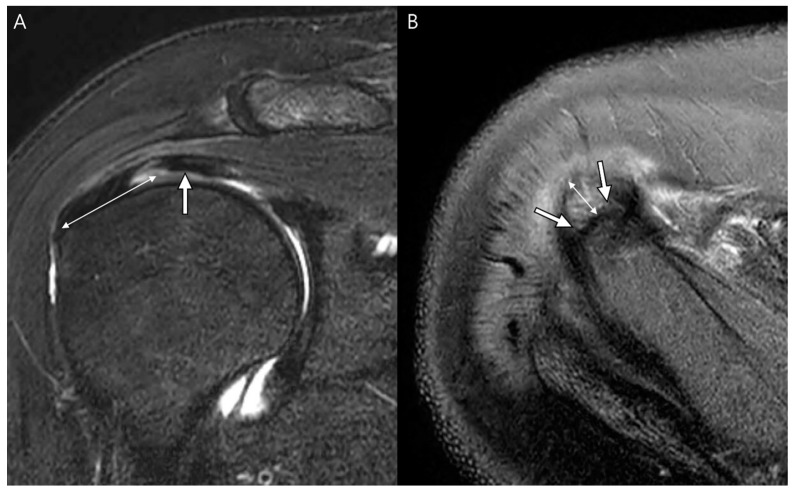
Rotator cable and crescent. (A) Oblique coronal fat-suppressed T2-weighted image and (B) axial fat-suppressed T2-weighted image demonstrate the low-signal thick bundle of rotator cable (thick arrow) perpendicular to the rotator cuffs and the distal fibers of rotator cuffs from the rotator cable to the greater tuberosity of the humerus called the rotator crescent (arrow bar).
